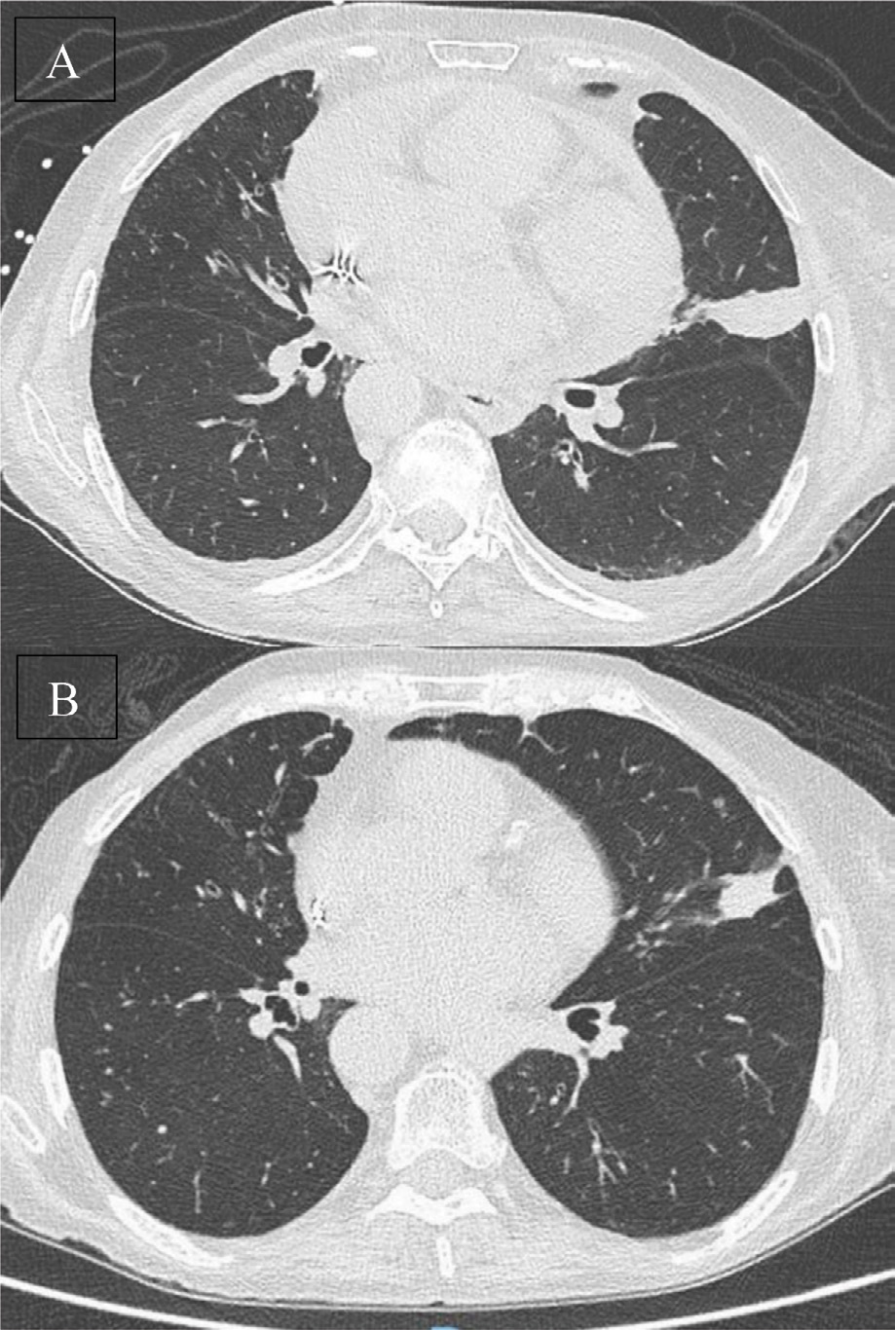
Axial CT images of the treated pulmonary carcinoid tumor at 1‑month (A) and 5‑month (B) follow‑up, showing sustained regression of the ablation zone without evidence of recurrence or new complications.
